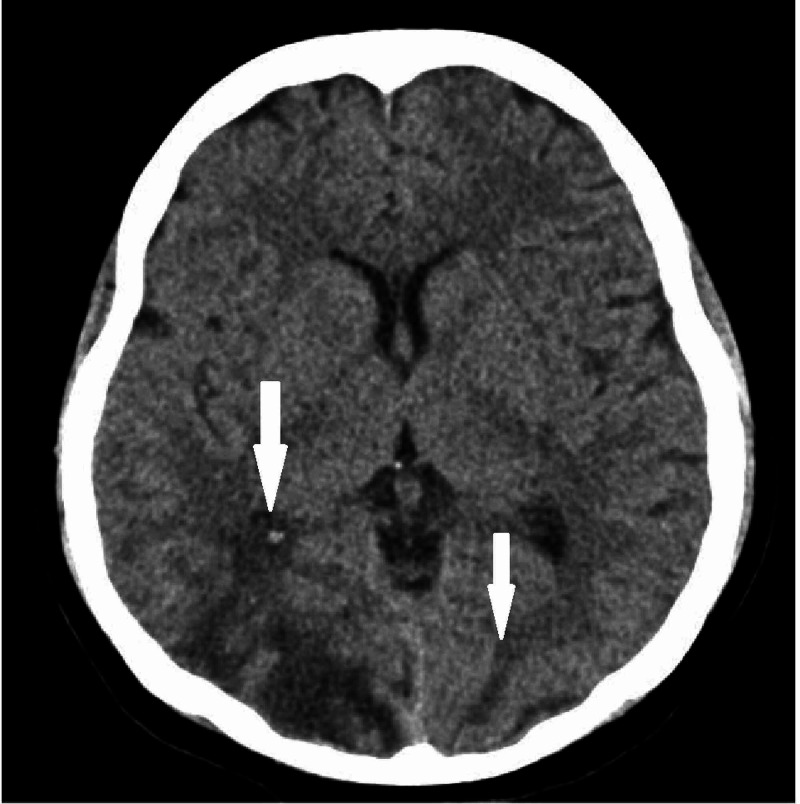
Computed tomography (CT) scan of the brain showed bilateral asymmetrical cortical and subcortical hypodense areas at bilateral occipital lobes (arrows), a finding consistent with posterior reversible encephalopathy syndrome (PRES).
